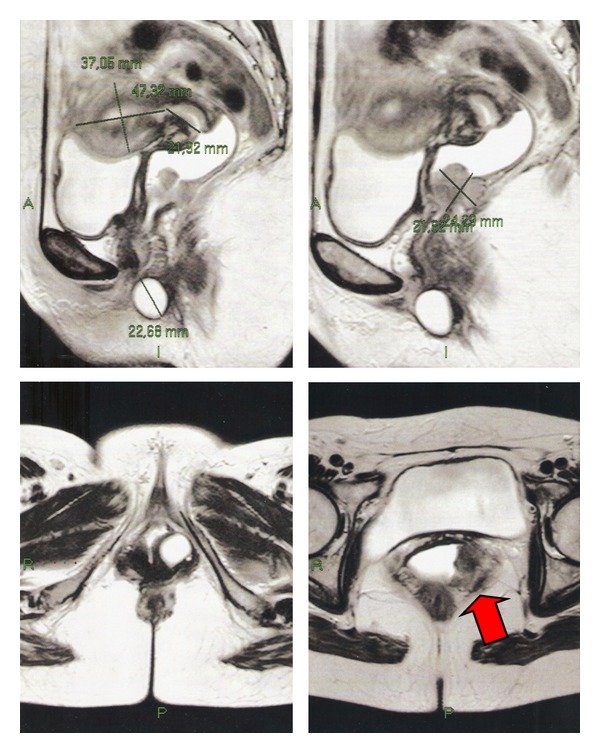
Magnetic resonance imaging: a solid mass in the middle third of the posterior wall of the vagina with invasion of left parametrium.
